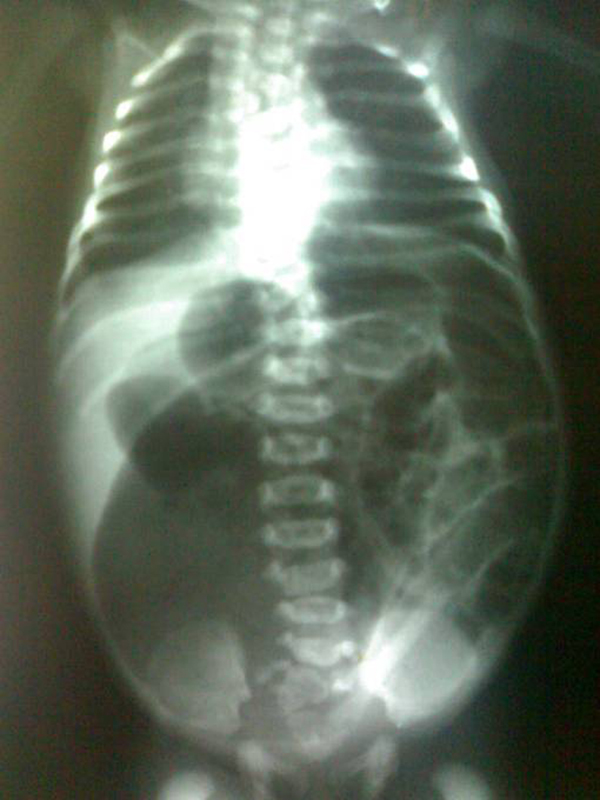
A supine abdominal radiograph showing large dilated bowel loop occupying more than half of the diameter of the abdomen.
